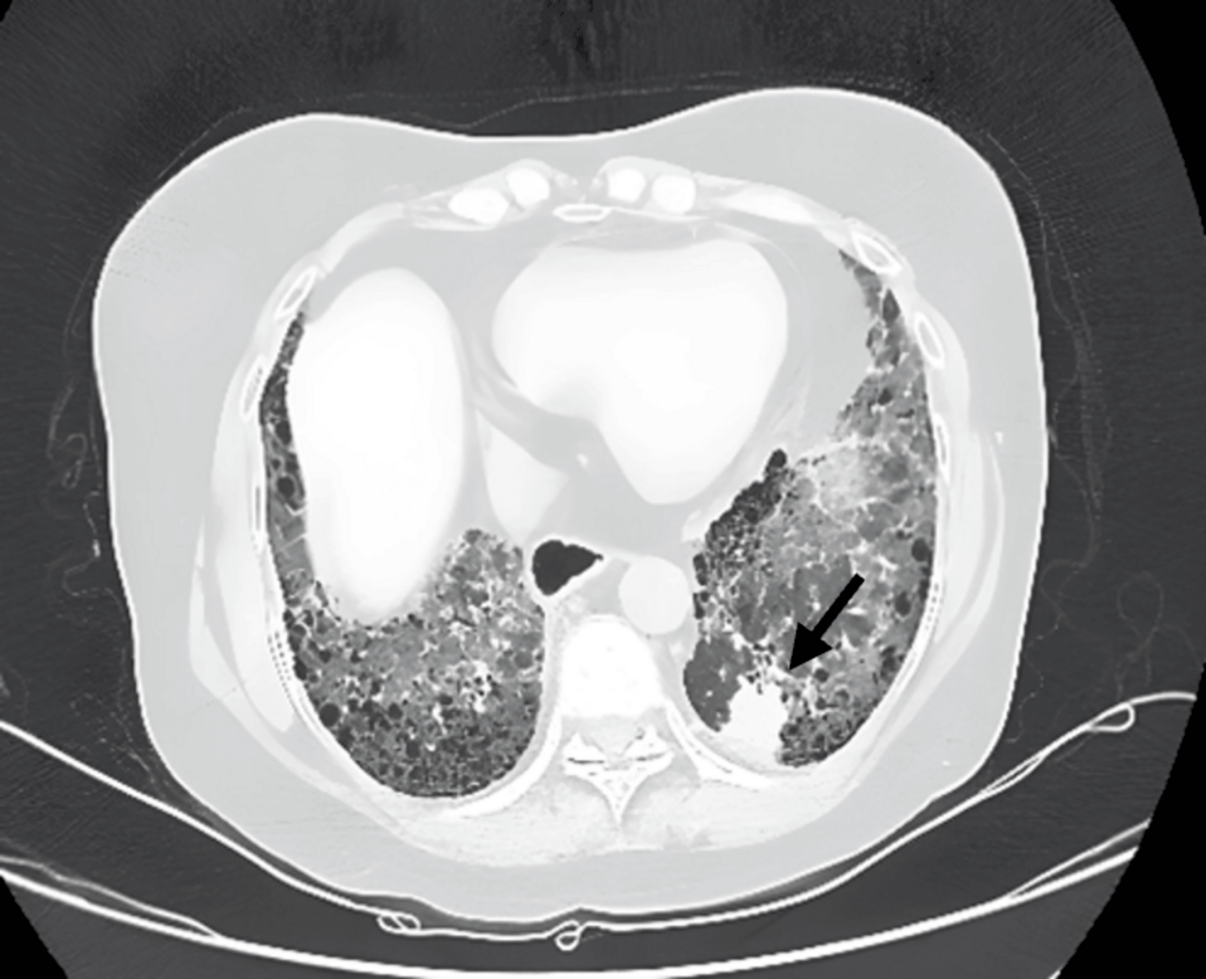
A follow-up CT of the chest shows a radiological progression of the interstitial lung disease (UIP), bilateral lower lobe subpleural honeycombing, reticular opacities, interlobular septal thickening, and an ill-defined, heterogeneously enhancing lesion in the left lower lobe posterobasal segment, measuring 2.5 x 2.6 cm
